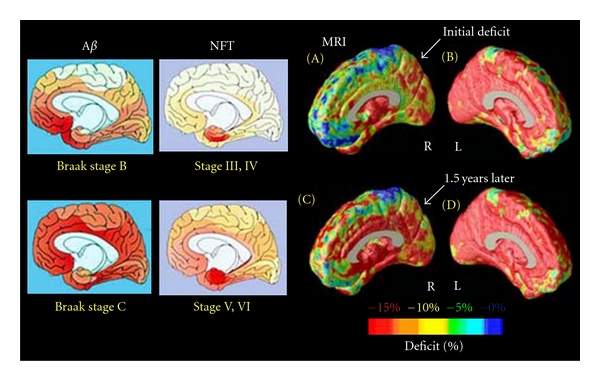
Gray matter deficits spread through the limbic system in moderate AD. Average maps of gray matter density in patients and controls were subtracted at their first scan (A and B) and at their followup scan 1.5 years later (C and D). Colors show the average percentage loss of gray matter relative to the control average. Profound loss engulfs the left medial wall (*>*15%; B and D). On the right, however, the deficits in temporoparietal and entorhinal territory (A) spread forward into the cingulate gyrus 1.5 years later (C). Limbic and frontal zones clearly show different degrees of impairment (C). MRI-based changes, in living patients, agree strongly with the spatial progression of *β*-amyloid (A*β*) and NFT pathology observed after mortem (Braak Stages B, C, and III to VI), (adapted with permission from [37, 38]).
